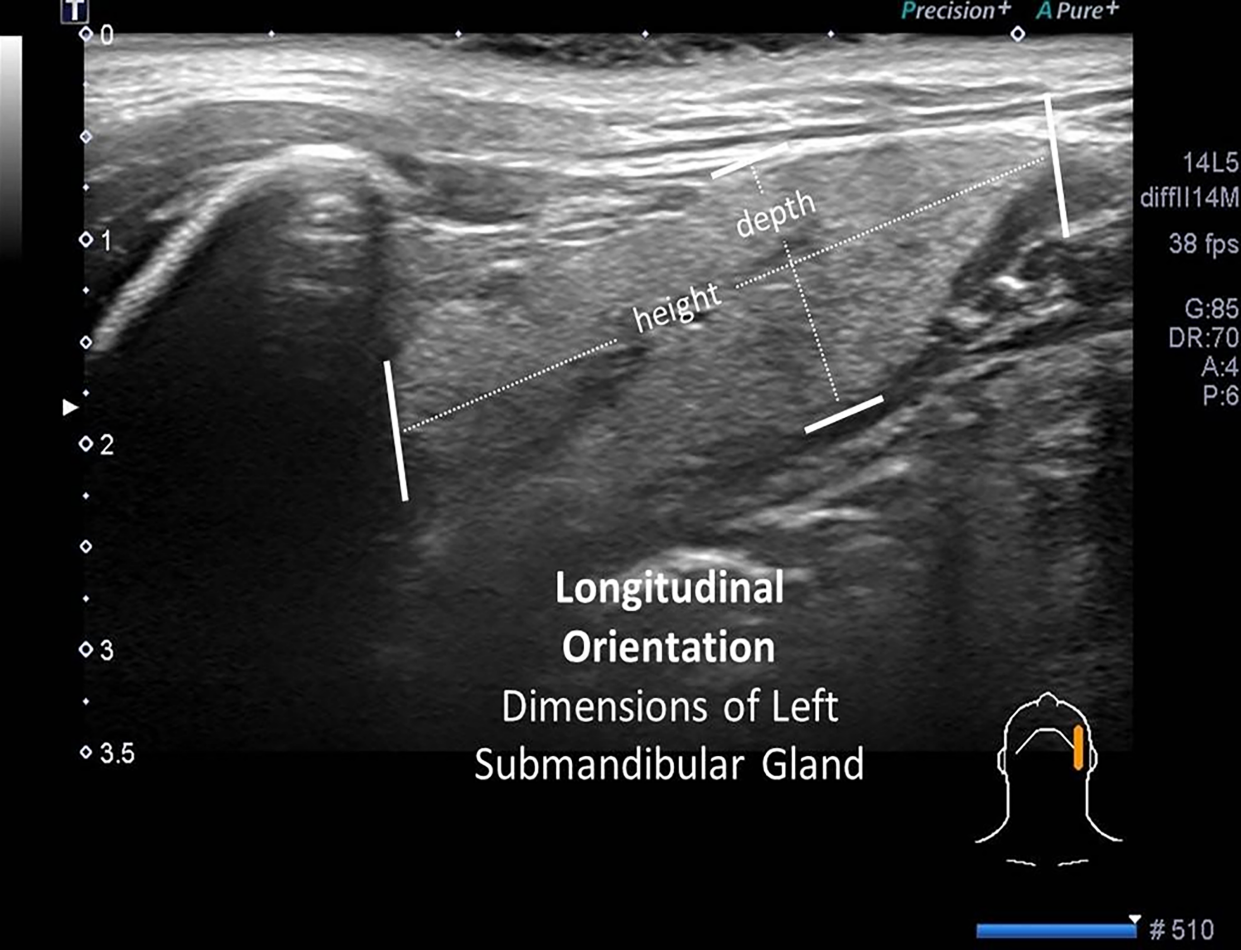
Longitudinal orientation of the 14–5 MHz linear ultrasound probe (with slight obliquity) identifies the height and depth of the left submandibular gland.
